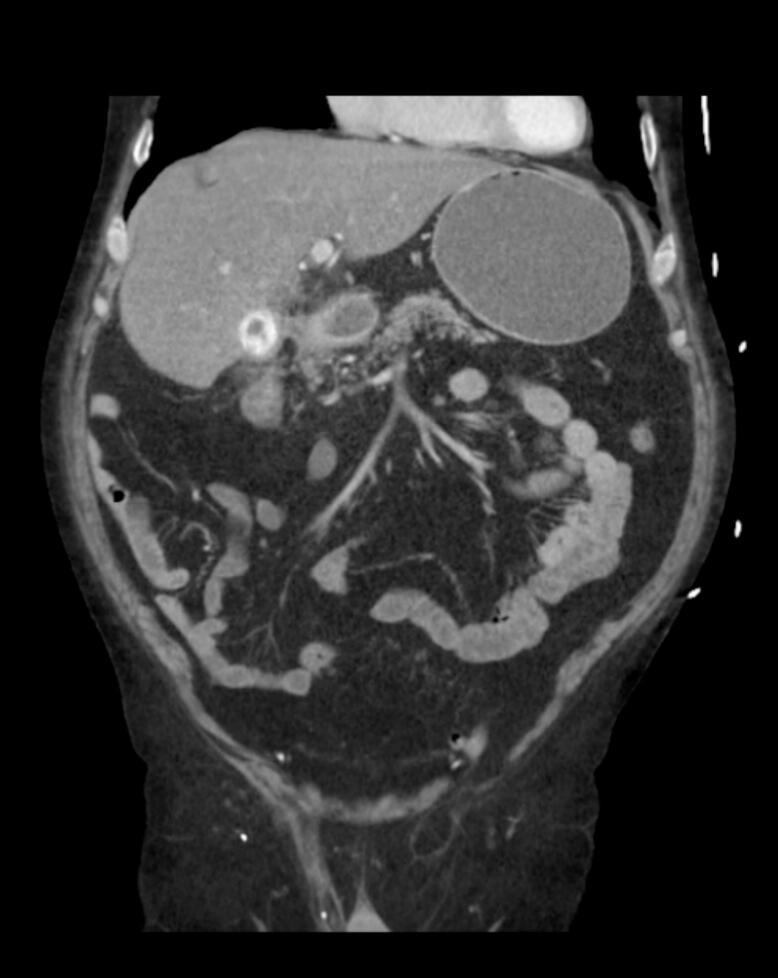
Patient 2's repeat abdominal and pelvic CT scan showing a large gallstone within the duodenum.
